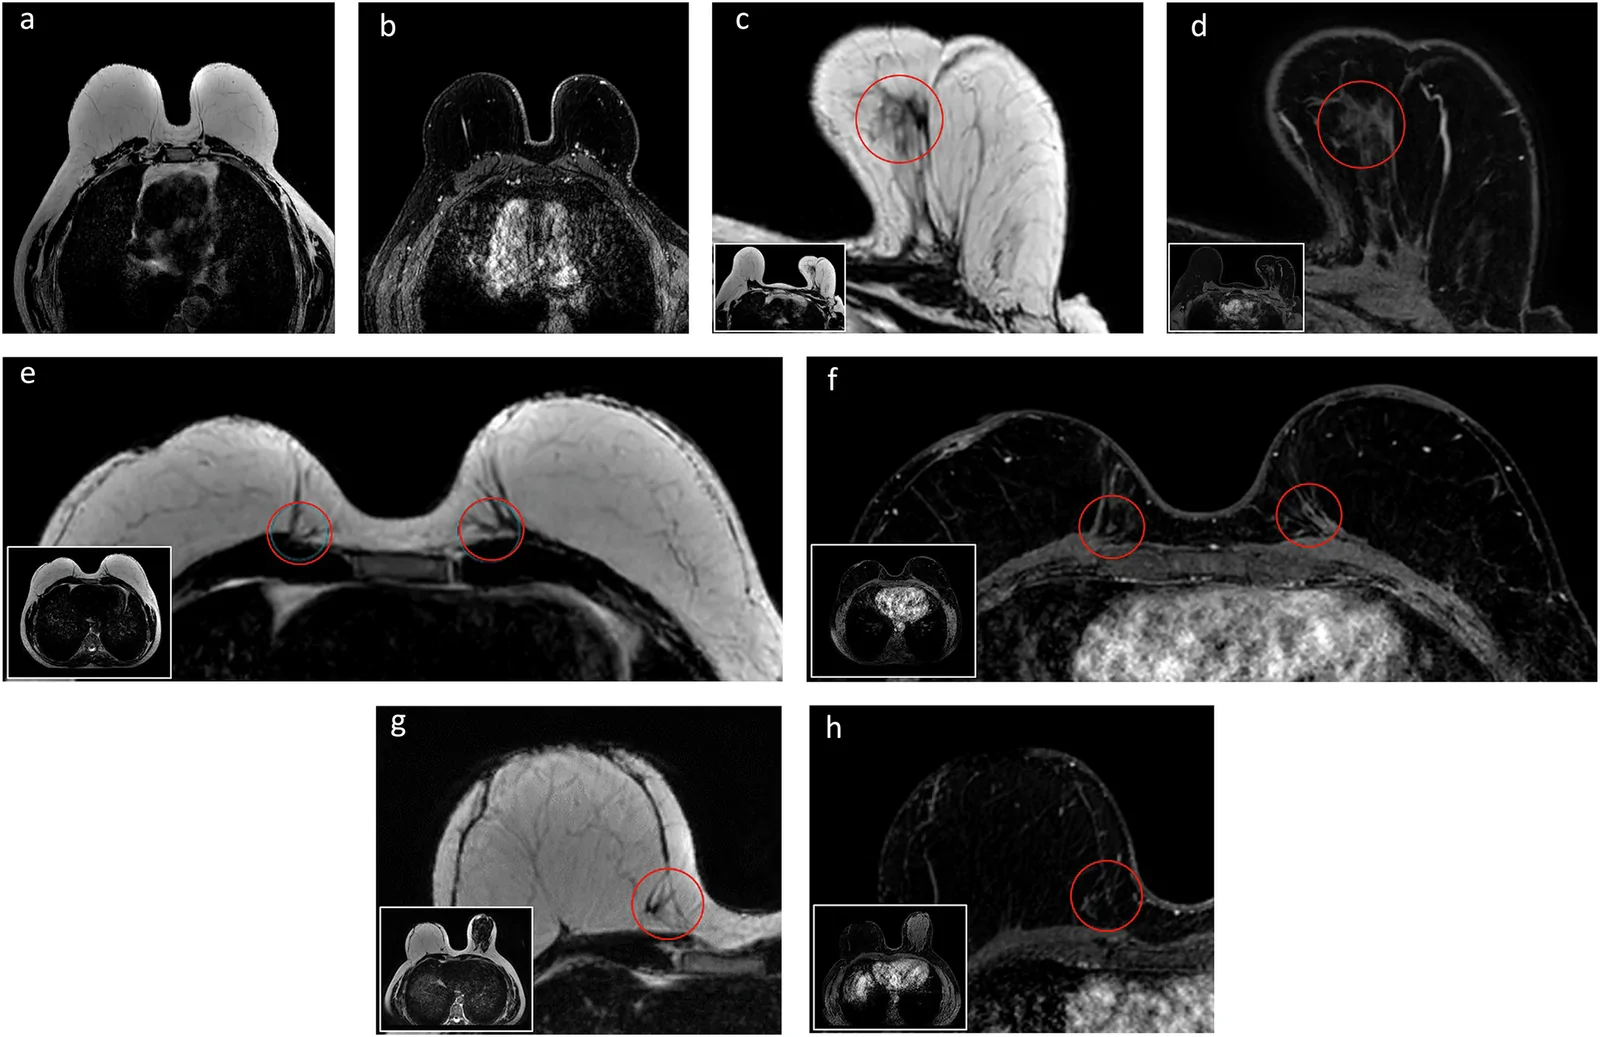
Breast MRI exams assessed for RFGT presence. RFGT is indicated by the red circle if applicable. a T2 and b T1 weighted breast MRI images of a patient with a bilateral delayed DIEP flap reconstruction, with a confidence score in consensus of 1, because there were no suspect areas for RFGT. c T2 and d T1 weighted breast MRI images of a patient with a unilateral delayed DIEP flap reconstruction after modified radical mastectomy, with a confidence score in consensus of 3, because of the area located in the upper inner quadrant of the breast. A score of 3 was given because the area could be the result of fibrosis with traction, as well as RFGT. e T2 and f T1 weighted breast MRI images of a patient with bilateral nipple sparing mastectomy and immediate DIEP flap reconstruction, where RFGT with a confidence score of 4 was located bilaterally in the upper inner quadrants of both breasts. g T2 and h T1 weighted breast MRI images of a patient with unilateral modified radical mastectomy and immediate DIEP flap reconstruction, where RFGT with a confidence score of 5 was located in the upper inner quadrant of the breast
